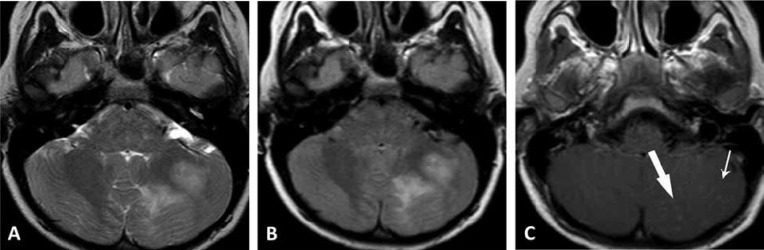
Repeat MRI fourteen months later. (A) Axial T2-weighted image, (B) axial FLAIR image showing new, extensive, patchy areas of T2 prolongation in the white matter of the left cerebellar hemisphere and (C) post contrast axial T1-weighted image demonstrating persistent mild diffuse leptomeningeal enhancement (small arrow) with new areas of nodular parenchymal enhancement (large arrow) in the left cerebellar hemisphere.
